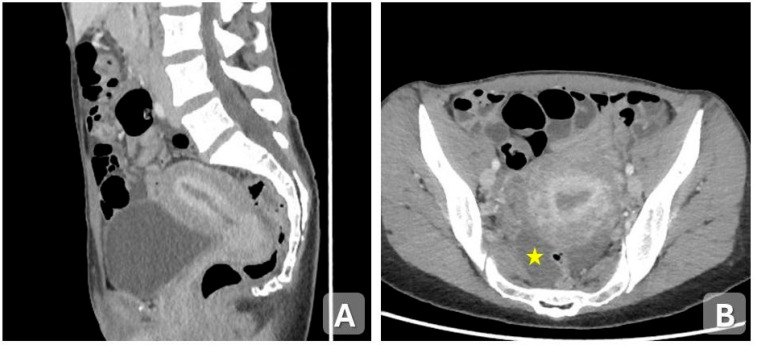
Acute endometritis in a 60-year-old woman with pelvic pain and a low-grade fever for 5 days. (A) Axial and (B) sagittal contrast-enhanced CT images show an enlarged, globular uterus with distension of the endometrial cavity containing hypodense fluid. There is enhancement of the endometrial lining after contrast administration. A large amount of free pelvic fluid is present (star).
